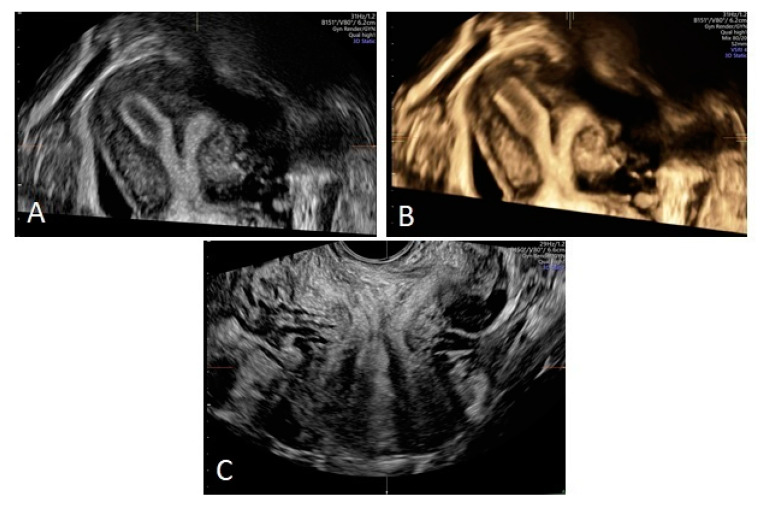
3D ultrasound of complete septate uterus. (A,B) Two uterine bodies divided by a septum; (C) Two uterine cervices.
